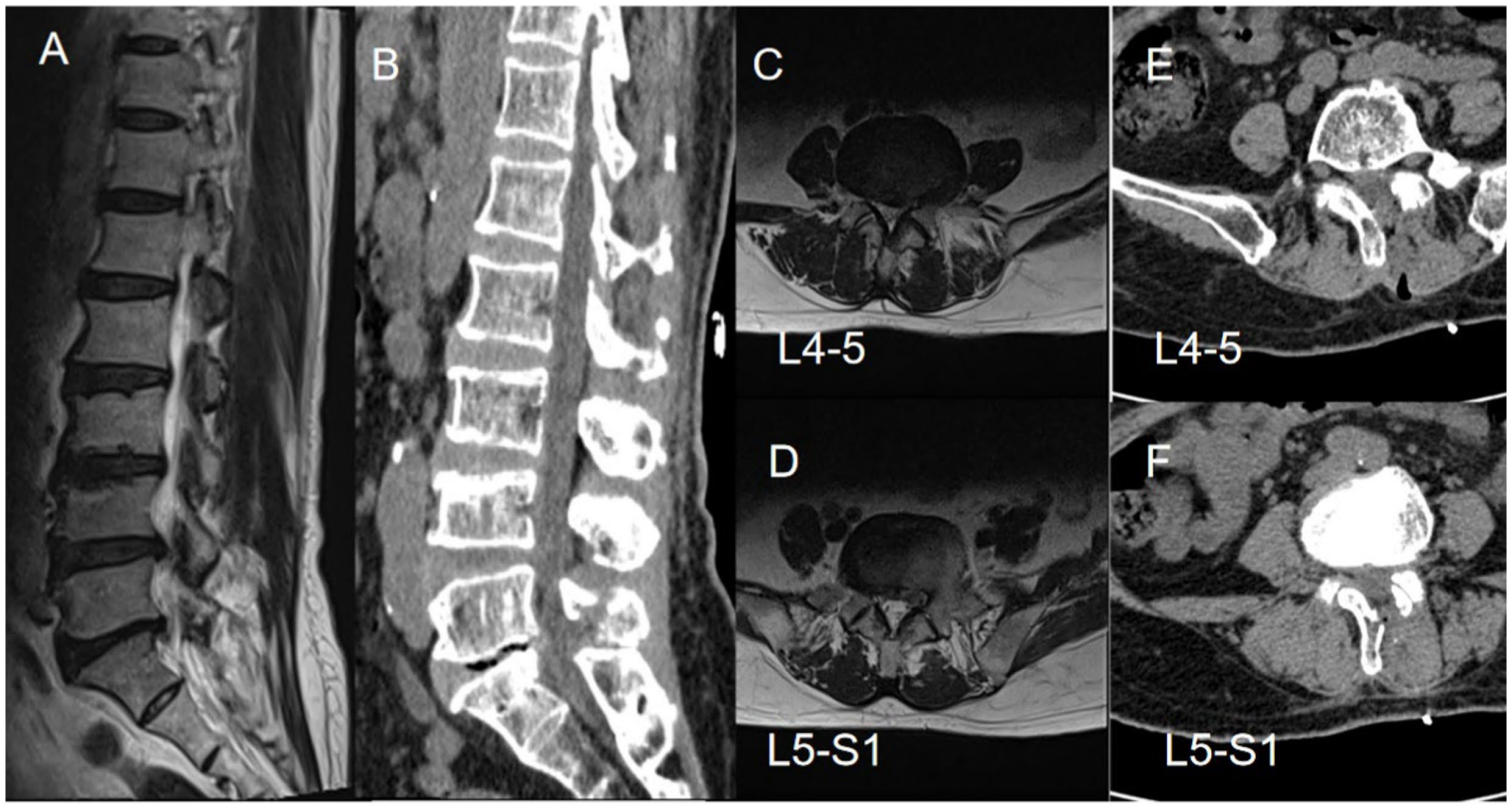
A 70-year-old female patient with disc herniation at the L4-5 and L5-S1 levels presented with pain and numbness in the lateral and posterior regions of the left lower leg, along with pain in the lateral aspect of the right calf. Preoperative axial and sagittal MR images revealed significant spinal canal stenosis at the L4-5 and L5-S1 levels (A–D). Subsequent postoperative three-dimensional CT scans displayed an increase in the width of the spinal canal following the surgical intervention (E,F).
